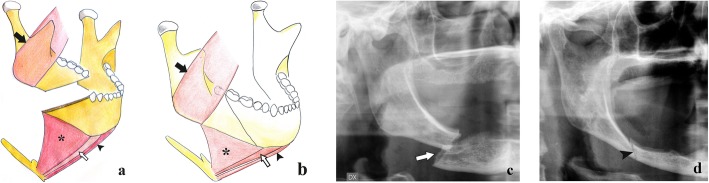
Horizontal branch fractures. a Unfavourable fracture. Picture showing a fracture with a downward and posterior direction. The bone fragments are misaligned by the action of the masseter muscle (black arrow) that pulls the distal bone fragment upwards, and the mylohyoid (asterisk), geniohyoid (white arrow), and anterior belly of digastric (black arrowhead) muscles that pull the mesial bone fragment downwards. b Favourable fracture. Picture showing a fracture with a downward and anterior direction. The bone fragments impact each other with no displacement. c, d Cropped panoramic radiographs in toothless patients with unfavourable (arrow) and favourable (arrowhead) horizontal branch fractures
